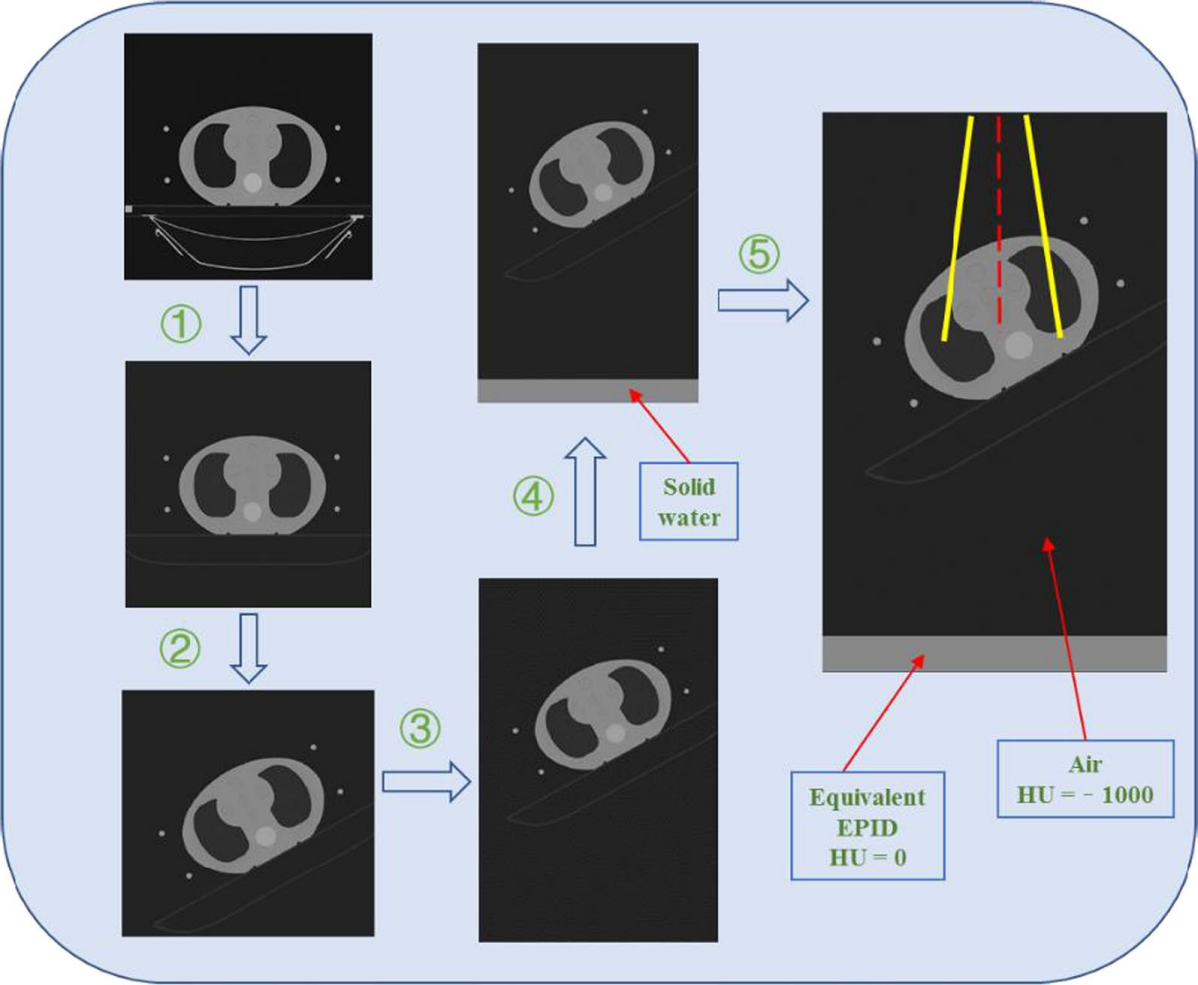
The pre-processing process of the plan CT when the gantry angle is 30°
